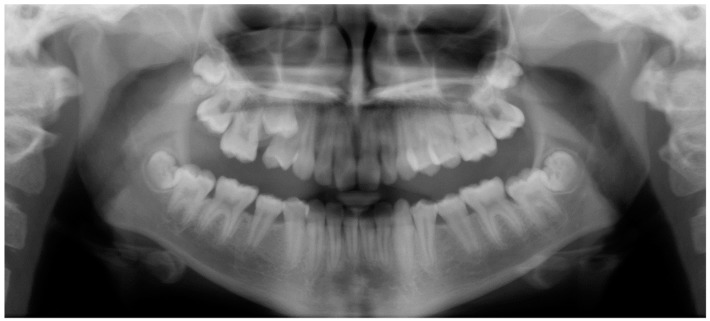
A conventional 2D panoramic radiograph showing limited information about the location of the impacted maxillary right second premolar.
